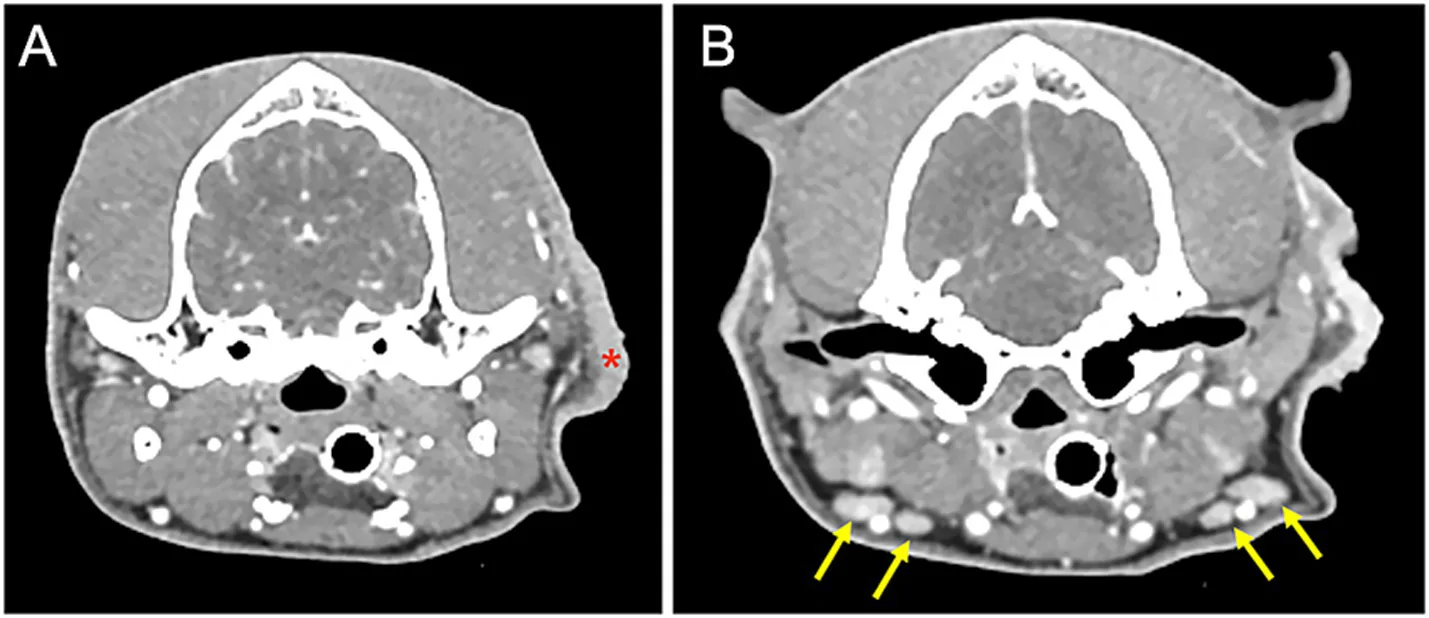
Computed tomographic images of the facial lesion and regional lymph nodes. (A) Computed tomography (CT) image showing focal cutaneous thickening (*) up to 5.0 mm at the level of the left ear tragus. The lesion exhibited isoattenuating enhancement relative to the surrounding cutaneous tissue, with attenuation values measured in Hounsfield units (HU) (pre-contrast: 67 HU; arterial: 124 HU; portal: 197 HU; delayed: 215 HU). (B) Mild enlargement of regional lymph nodes, including bilateral mandibular (yellow arrow, right: 4.1 mm, left: 4.7 mm), retropharyngeal (right: 4.0 mm, left: 4.6 mm), and superficial cervical nodes (right: 7.7 mm, left: 7.5 mm), was observed on CT.
